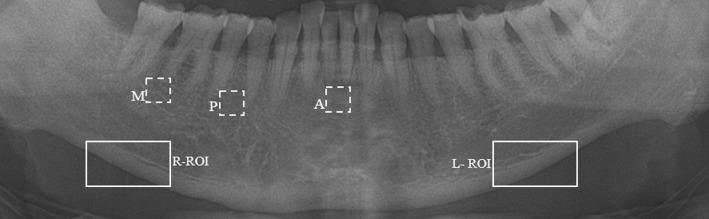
Digital panoramic radiograph with right (R) and left (L) regions of interest (ROI) for mandibular cortical width measurements and molar (M), premolar (P) and anterior (A) regions of trabecular bone for fractal dimension measurements.
